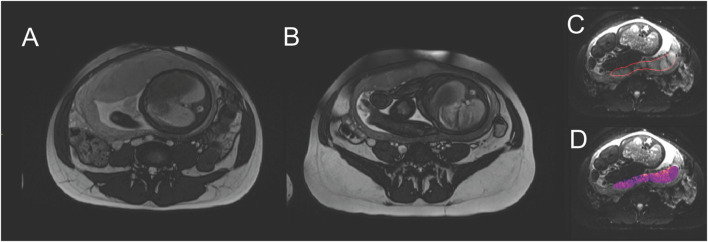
Example of placental MRI modalities. Comparing central slices through the placenta, (A) is a normal pregnancy (fetal abdominal diameter 96.5 mm) at 37 weeks gestation, with placental thickness of 41.2 mm. (B) is FGR (fetal abdominal diameter 79.2 mm, birthweight 1.4 centile) at 37 weeks gestation, with placental thickness of 21.8 mm. Placentae in FGR pregnancies are typically described as smaller and appearing darker and more heterogenous in MRI. (C) shows identification of a placental region of interest. (D) shows a parameter map of apparent diffusion coefficient, a parameter that relates to blood movement, and is typically extracted from MRI studies.
